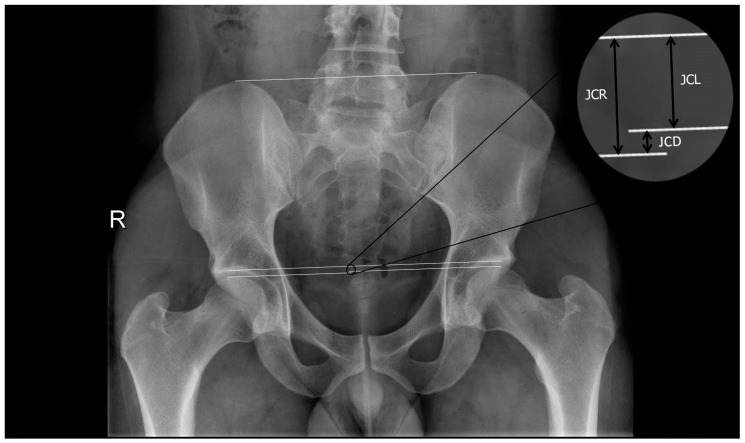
X-rays to quantify difference in the joint clearance. JCD: Joint clearance discrepancy; JCL: Joint clearance left; JCR: Joint clearance right.
